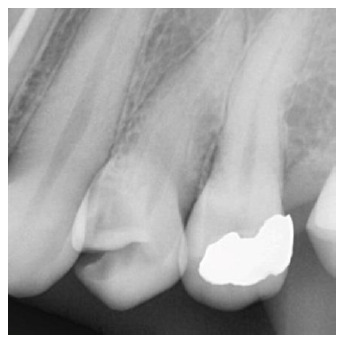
Pre-operative periapical radiograph of the left maxillary first premolar at the time of referral
